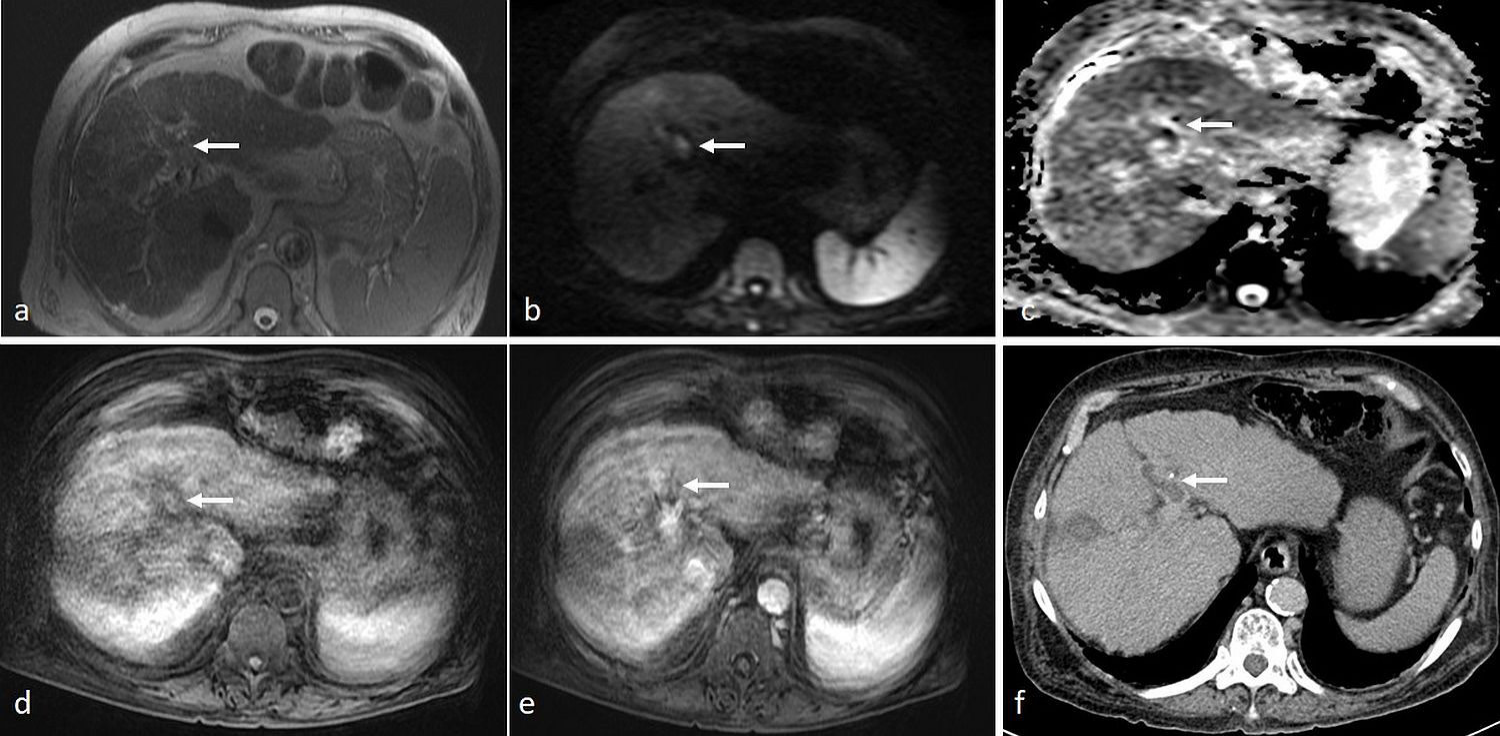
A 79-year-old gentleman underwent prior surgical resection and radiofrequency ablation procedures for recurrent HCC. MRI was performed with intravenous administration of Primovist. (a) On axial *T*
